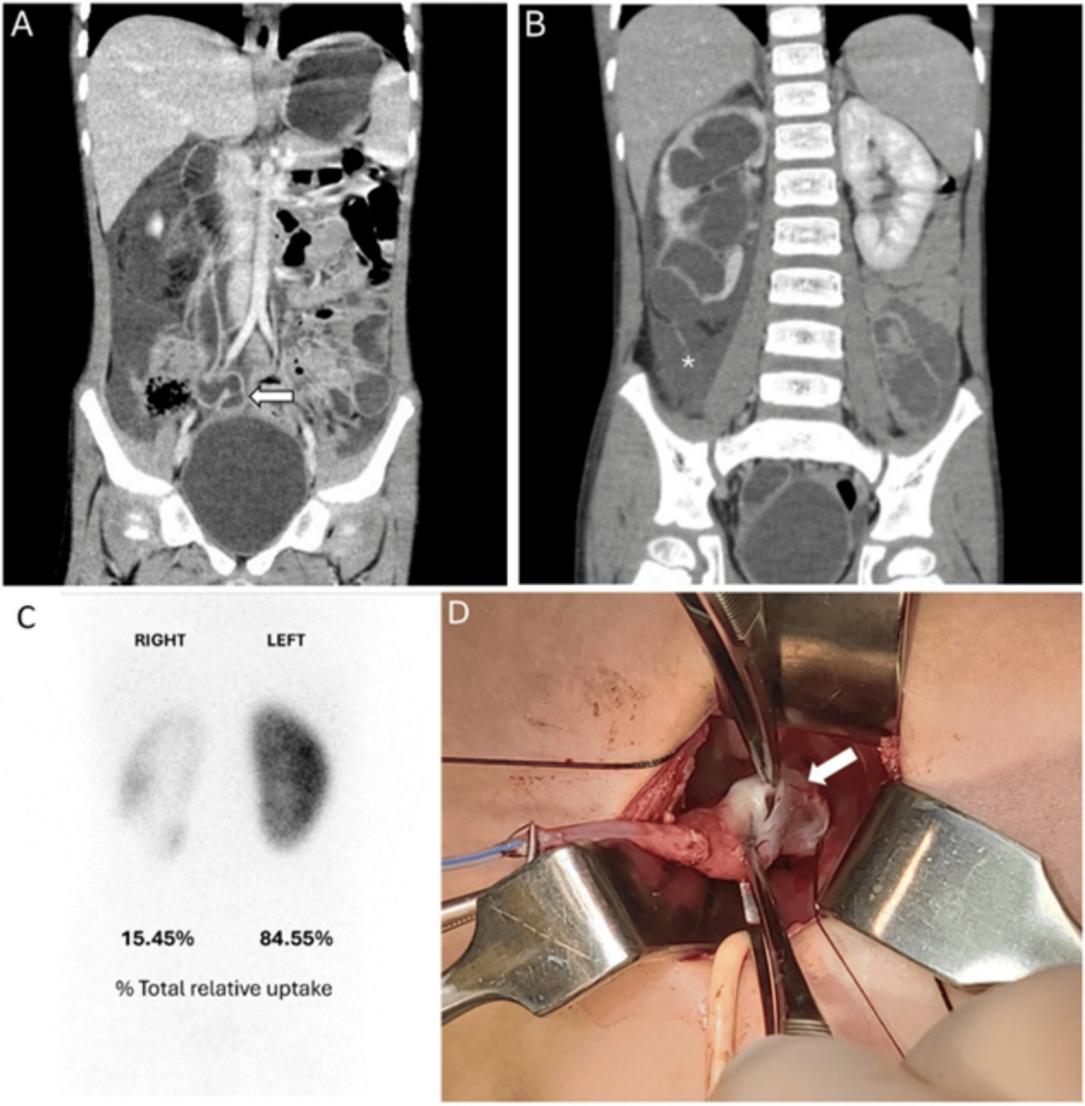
Imaging and Intraoperative Evidence of Our Case (A) Abdominal computed tomography showing significant right-sided hydronephrosis and hydroureter (arrow) (B) Abdominal computed tomography showing ascites localized to the right side (asterisk) (C) Dimercaptosuccinic acid kidney scintigraphy demonstrating severely impaired right kidney function (16%) and preserved left kidney function (84%) (D) Intraoperative image showing rupture in the distal right ureter (arrow)
